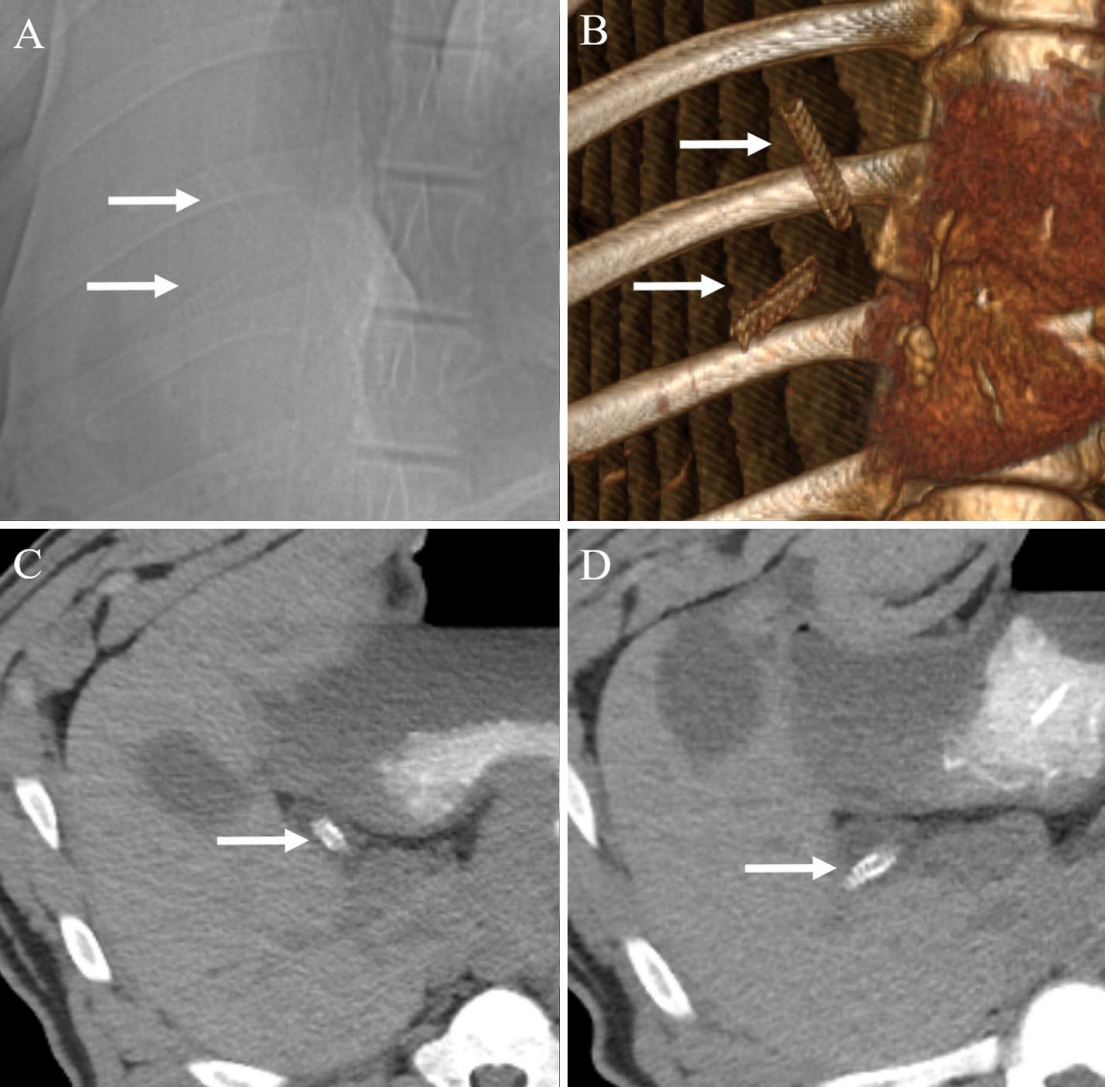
Two-month follow-up CT scan images showing stent fracture. (A) Scout image and (B) 3D-volume rendered image shows complete fracture of the stent (arrows). (C,D) Axial images also shows two fragments of fractured stent (arrows) within the common bile duct without any complications such as biliary obstruction or bile leakage.
